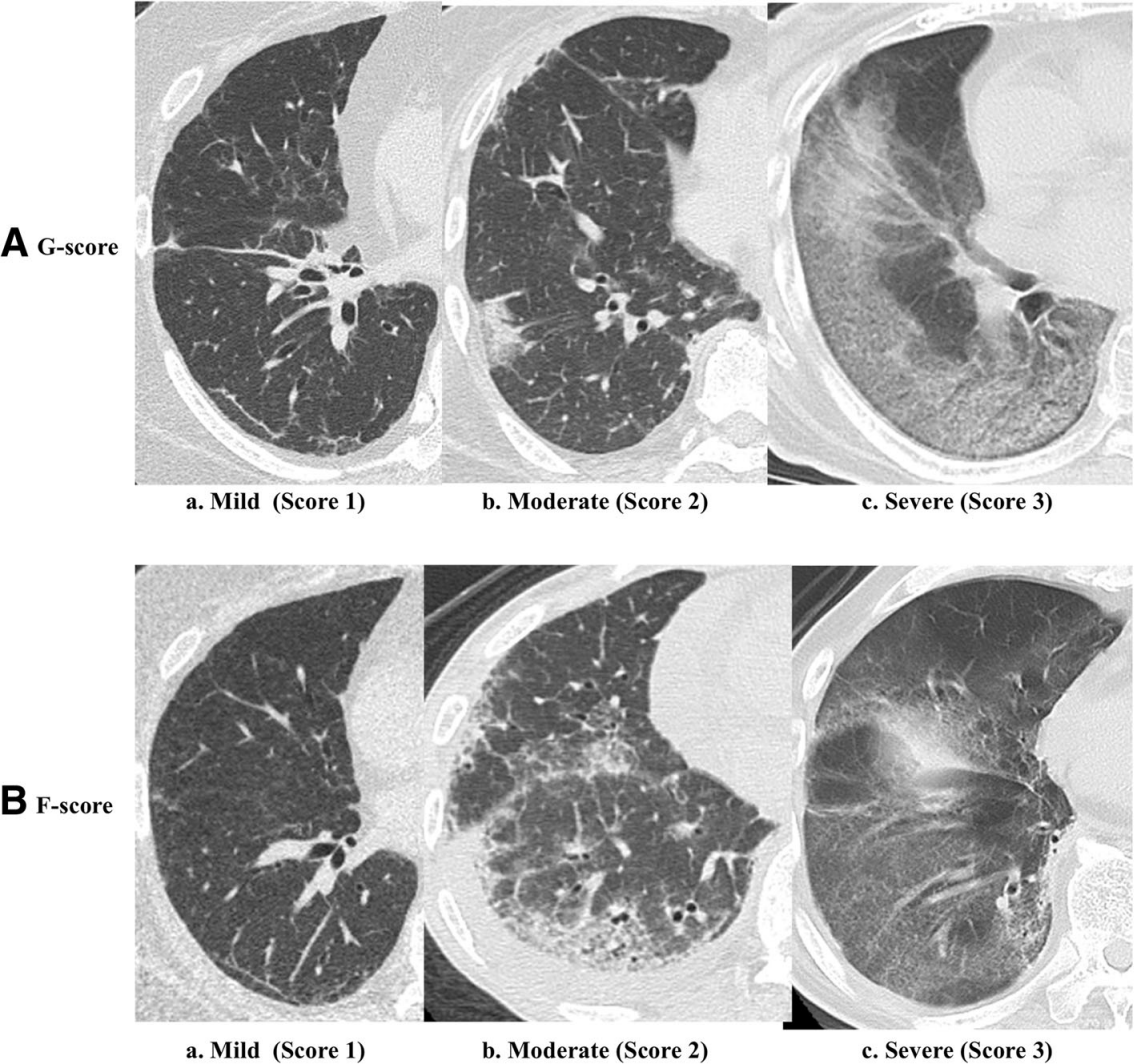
Assessment of rapidly progressive interstitial lung disease (RP-ILD) by computed tomography (CT) scores. A Assessment of RP-ILD by CT ground glass opacity (GGO) scores (G-scores): (a) thin-section CT scan shows small areas with GGO compared with normal parenchyma at the right lower lobe (mild GGO = 1); (b) CT scan shows extensive GGO that could be easily identified when compared with the normal parenchyma at the right lower lobe (moderate GGO = 2); (c) thin-section CT scan shows areas with diffuse GGO at the right lower lobe (severe GGO = 3). B Assessment of RP-ILD by CT fibrosis scores (F-scores): (a) thin-section CT scan shows areas with thickened interlobular septum or predominant peripheral fibrosis (mild fibrosis = 1). (b) CT scan shows extensive fibrosis that could be easily identified when compared with normal parenchyma at the right lower lobe (moderate fibrosis = 2), moderate fibrosis and bronchiolectasis. (c) thin-section CT scan shows areas with diffuse fibrosis at the right lower lobe (severe fibrosis = 3). Note honeycombing, bronchiectasis, peribronchovascular thickening, and subpleural cysts
